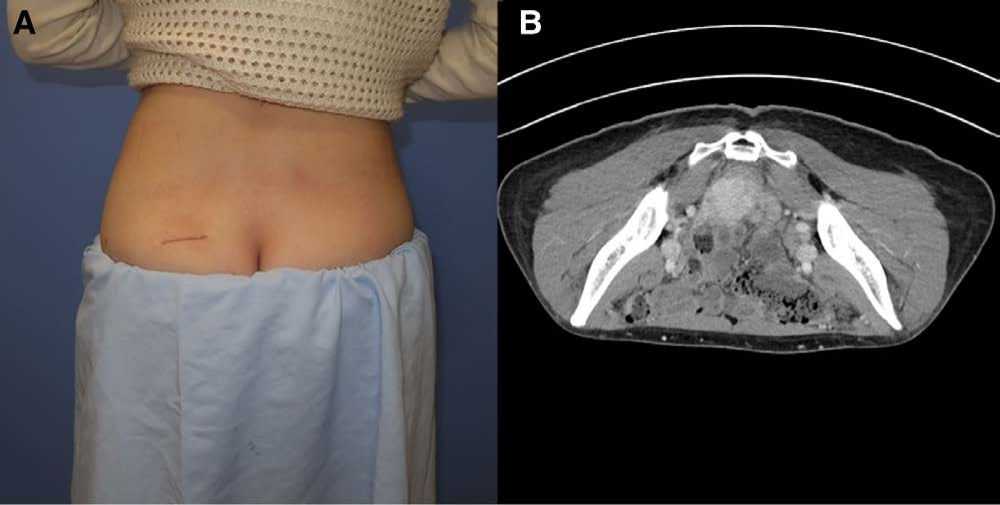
Clinical photograph and CT scan at the 18-month follow-up, demonstrating no evidence of recurrence or complications. CT = computed tomography.
